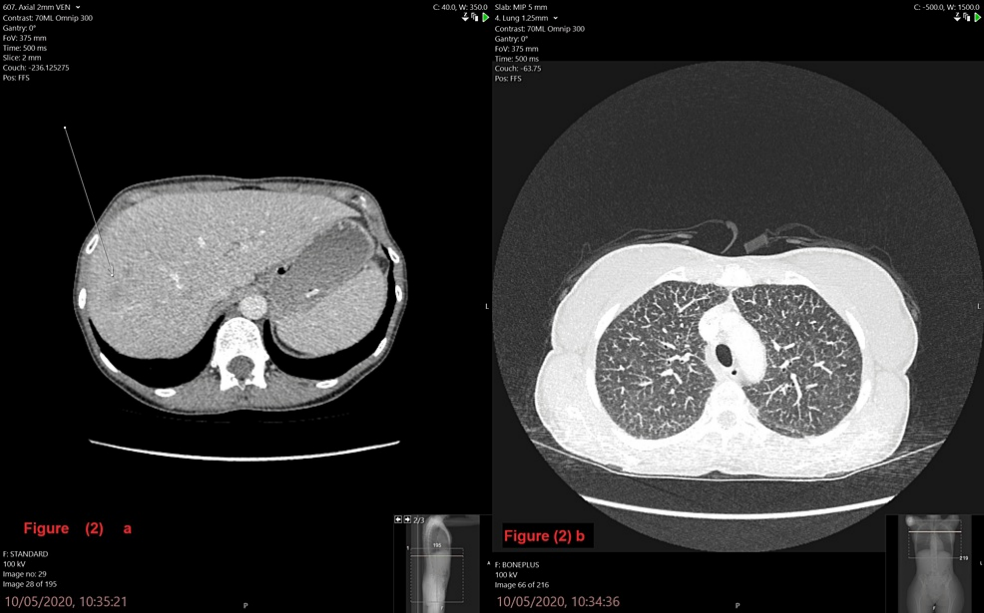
2a: CT scan of the patient's abdomen (axial view); 2b: CT scan of the chest (axial view) 2a: A CT of the abdomen (axial view) revealed multiple ill-defined hepatic lesions suggestive of metastasis vs. sarcoidosis, and the rest of the abdomen was normal; 2b: A CT scan of the chest (axial view) showed ground glass nodularity of the upper lobes and minor fissure nodules with no lymph node enlargement. These findings are most probably caused by sarcoidosis.
